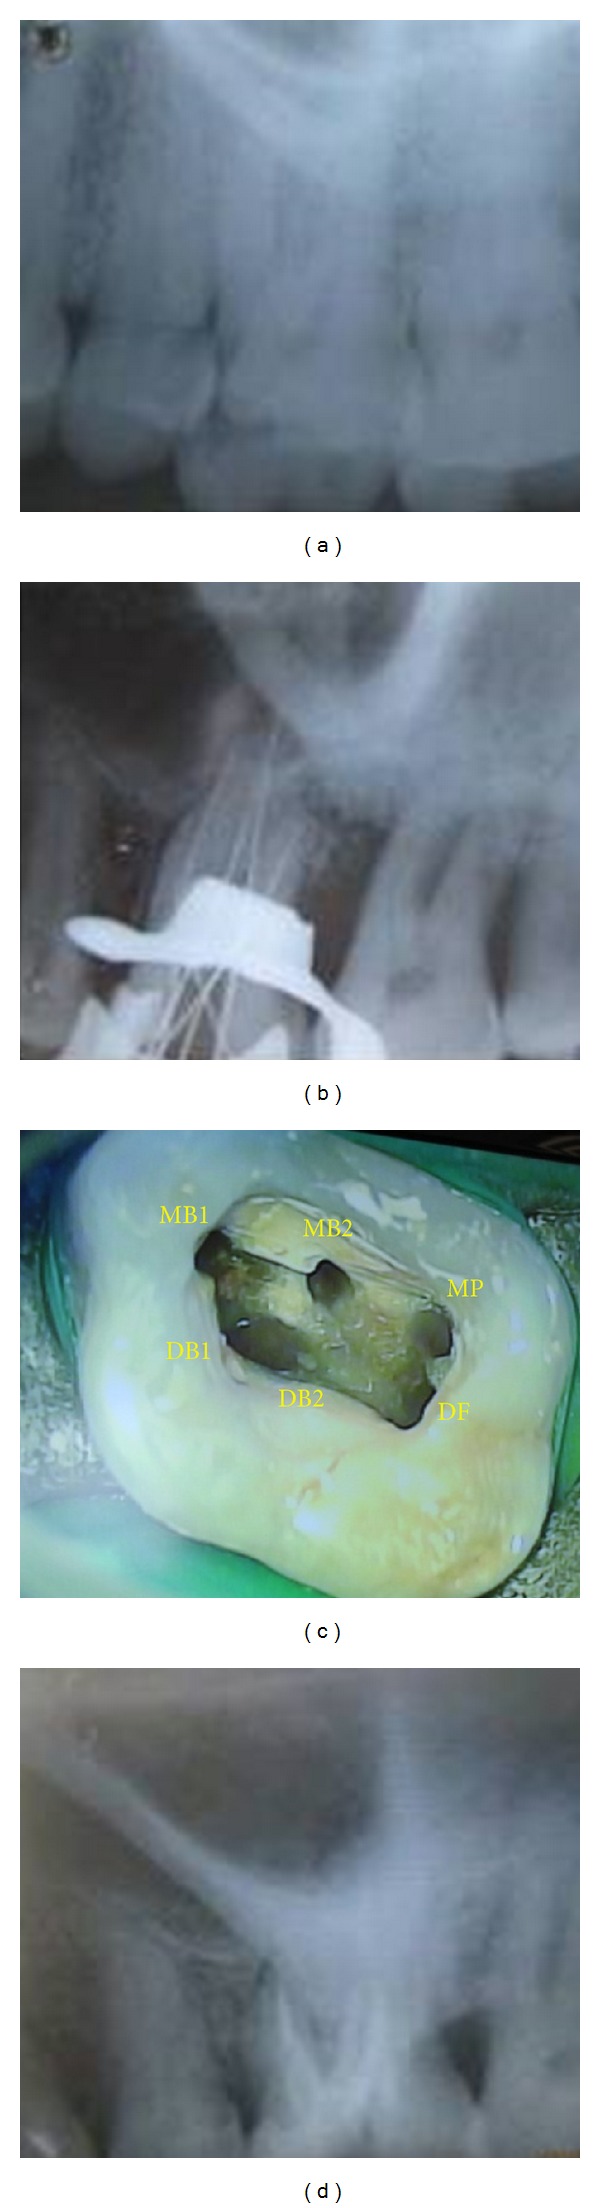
(a) Preoperative radiograph. (b) Working length radiograph showing multiple canals. (c) Access opening of the tooth showing multiple orifices. (d) Postobturation radiograph.
